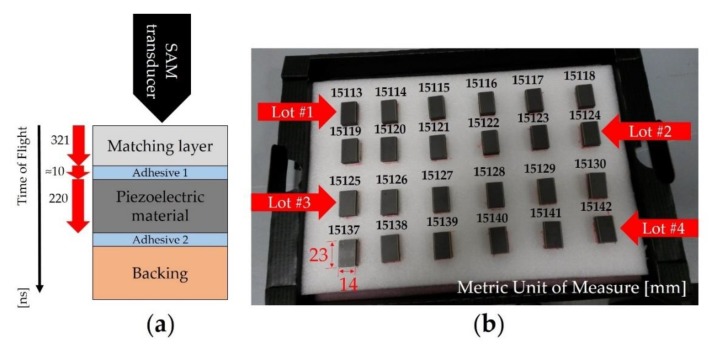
Samples selected for the SAM measurement campaign: (a) cross section of the samples under testing (not to scale) with the time of flights of the detected layers; (b) 24 acoustic stacks for ultrasonic transducer divided into 4 lots.
